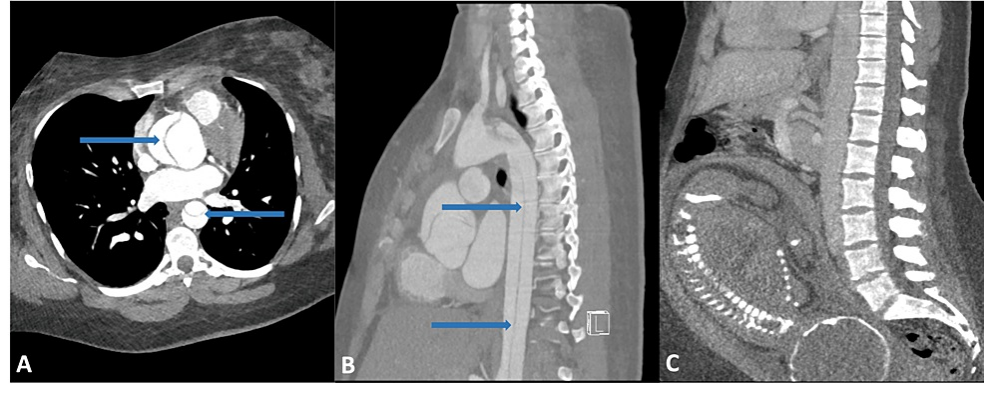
Computed tomography angiogram of the chest and abdomen (A) Axial image shows type A aortic dissection with a dissection flap (arrows) originating distal to the aortic root. (B) Sagittal image shows the dissection flap (arrows) continuing distally into the abdominal aorta. (C) Sagittal image shows the fetus in a cephalic position within the uterine cavity.
